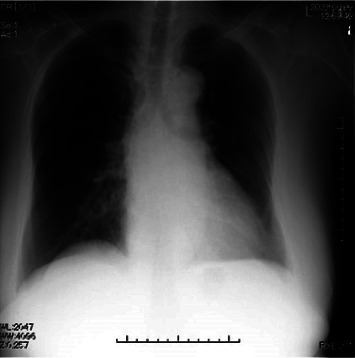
Preoperative chest radiograph.
